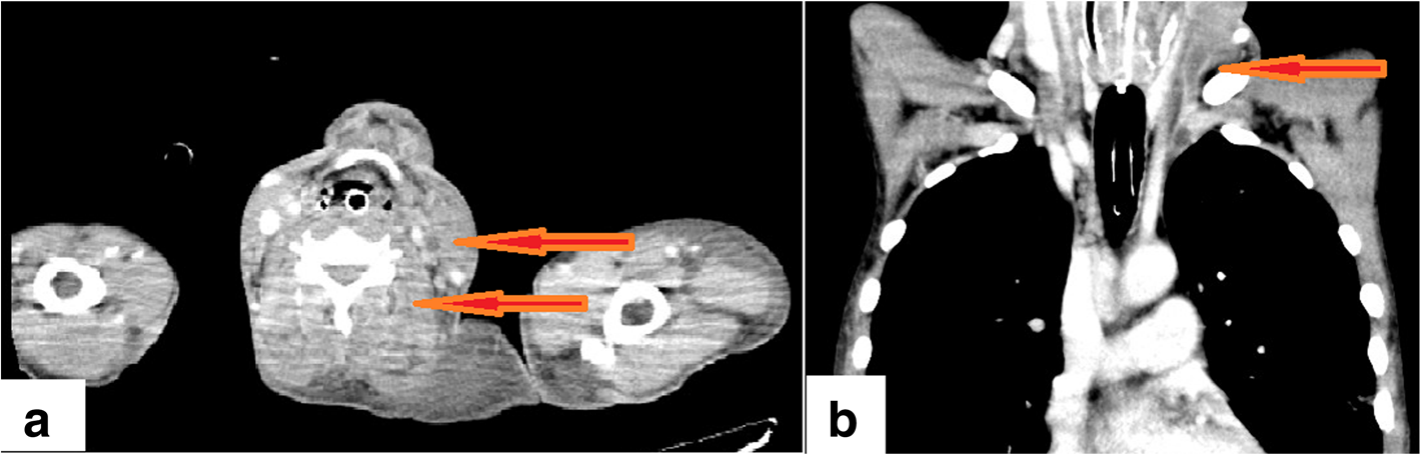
Imaging findings of a 44-year–old man who was diagnosed Lemierre’s syndrome. Contrast enhanced computed tomography on day 6 showed left internal jugular vein and vertebral vein thrombosis (arrow) (a. axial view, b. coronal view)
